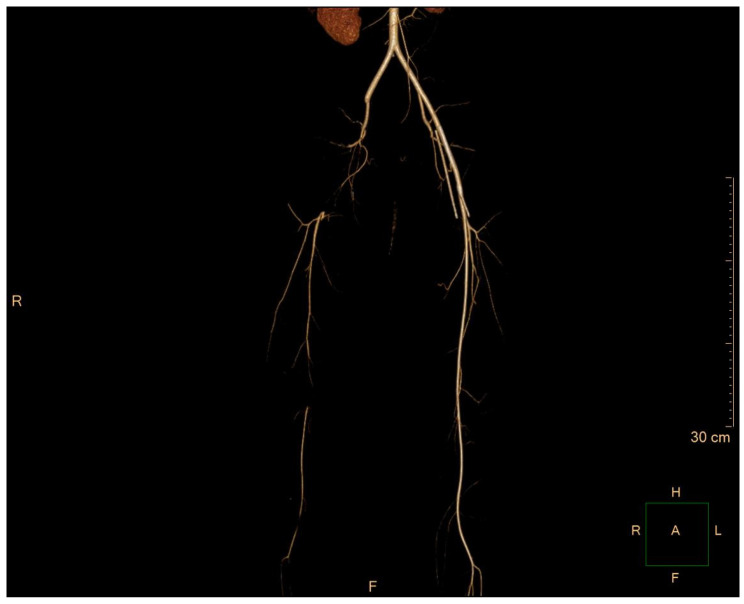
Lower-extremity angio CT was performed following the patient’s complaints of ischemic symptoms.
